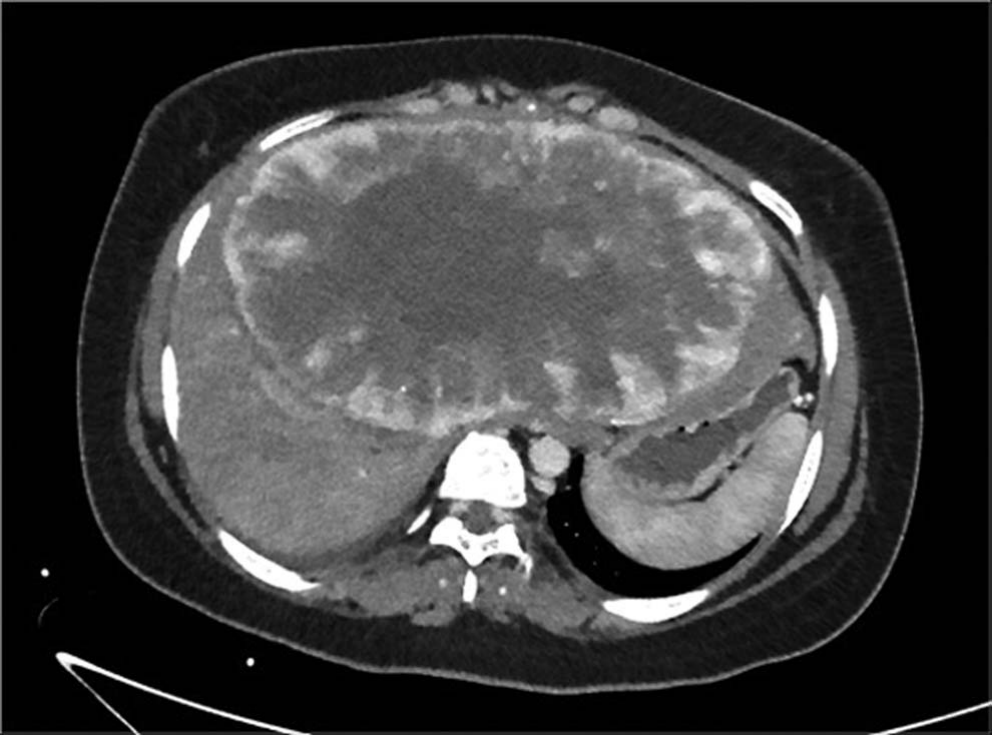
Computed tomography scan with contrast at the level of the upper abdomen shows a large (12 × 20 × 16 cm) heterogeneously enhancing mass centered in the left hepatic lobe and extending into the right lobe.
